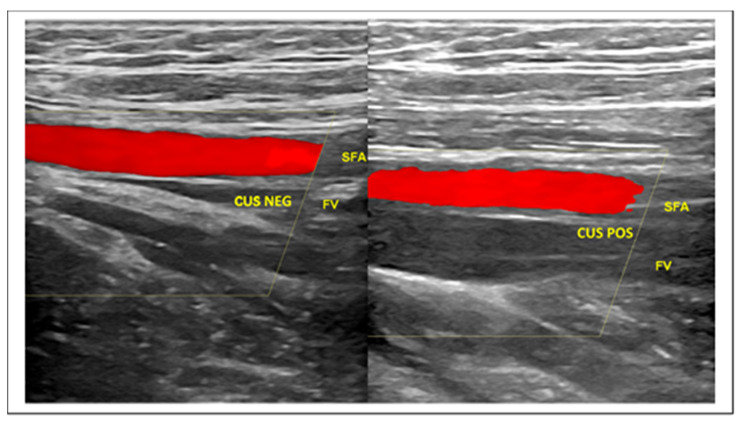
CUS maneuver for rapid identification of DVT. (Left) The real time compression by the ultrasound probe of the femoral vessels at the thigh determines the collapse of the femoral vein. CUS maneuver negative for DVT. (Right) The same maneuver in the case of femoral vein thrombosis does not demonstrate venous collapse. CUS maneuver positive for DVT. Legend: SFA—Superficial Femoral Artery; CUS NEG—Negative for thrombosis Compression Ultrasonography; FV—Femoral Vein; CUS POS—Positive for thrombosis Compression Ultrasonography.
